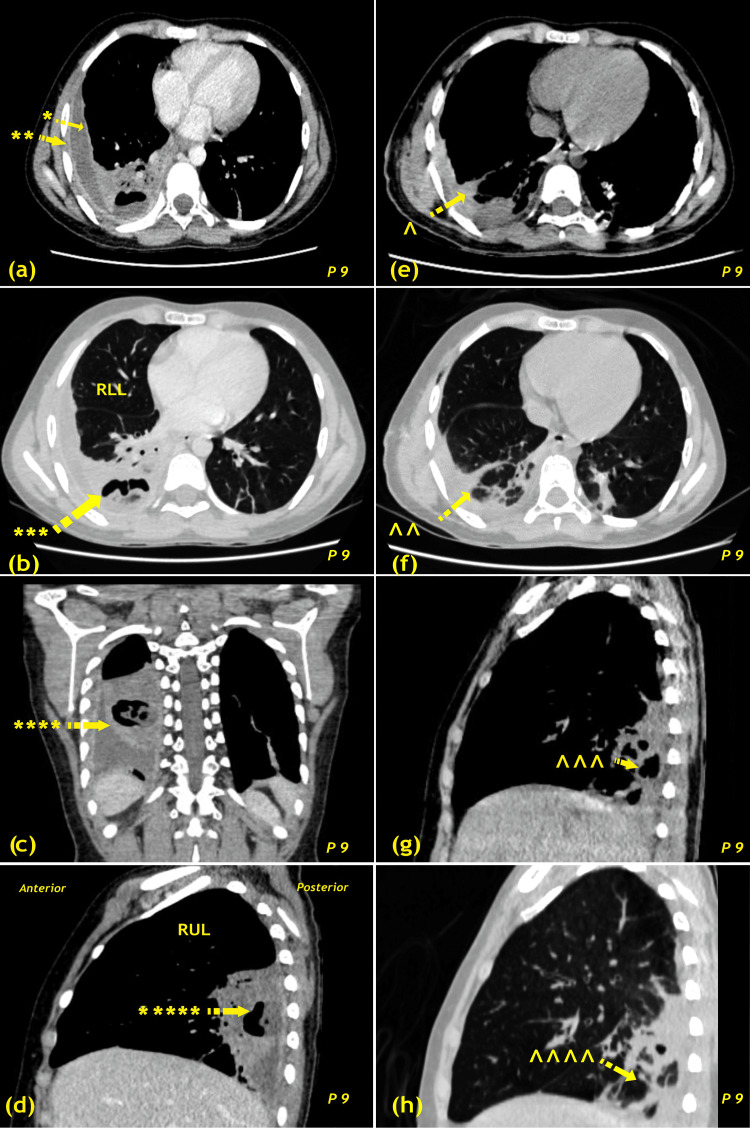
Pre- and postoperative CT scans Preoperative: (a) CECT thorax, axial mediastinal window shows right-sided loculated mild pleural effusion (*) with thickened enhancing visceral and parietal pleura (); (b) CECT thorax, axial lung window shows dense consolidation with internal air bronchogram and cavitation with air-fluid levels in the right lower lobe, suggestive of developing abscess (*); (c) CECT thorax, coronal mediastinal window shows right-sided loculated mild pleural effusion with thickened enhancing pleura and developing abscess (); (d) CECT thorax, sagittal mediastinal window shows similar findings (*). Postoperative: (e) HRCT thorax, axial mediastinal window shows significant reduction in pleural effusion with resolving consolidation (^); (f) HRCT thorax, axial lung window shows resolving consolidation (^^); (g) HRCT thorax, sagittal mediastinal window shows decrease in cavitation size (^^^); (h) HRCT thorax, sagittal lung window image (^^^^). CECT, contrast-enhanced CT; HRCT, high-resolution CT; P, patient; RLL, right lower lobe; RUL, right upper lobe; s/o, suggestive of Image credit: Viral B. Patel
